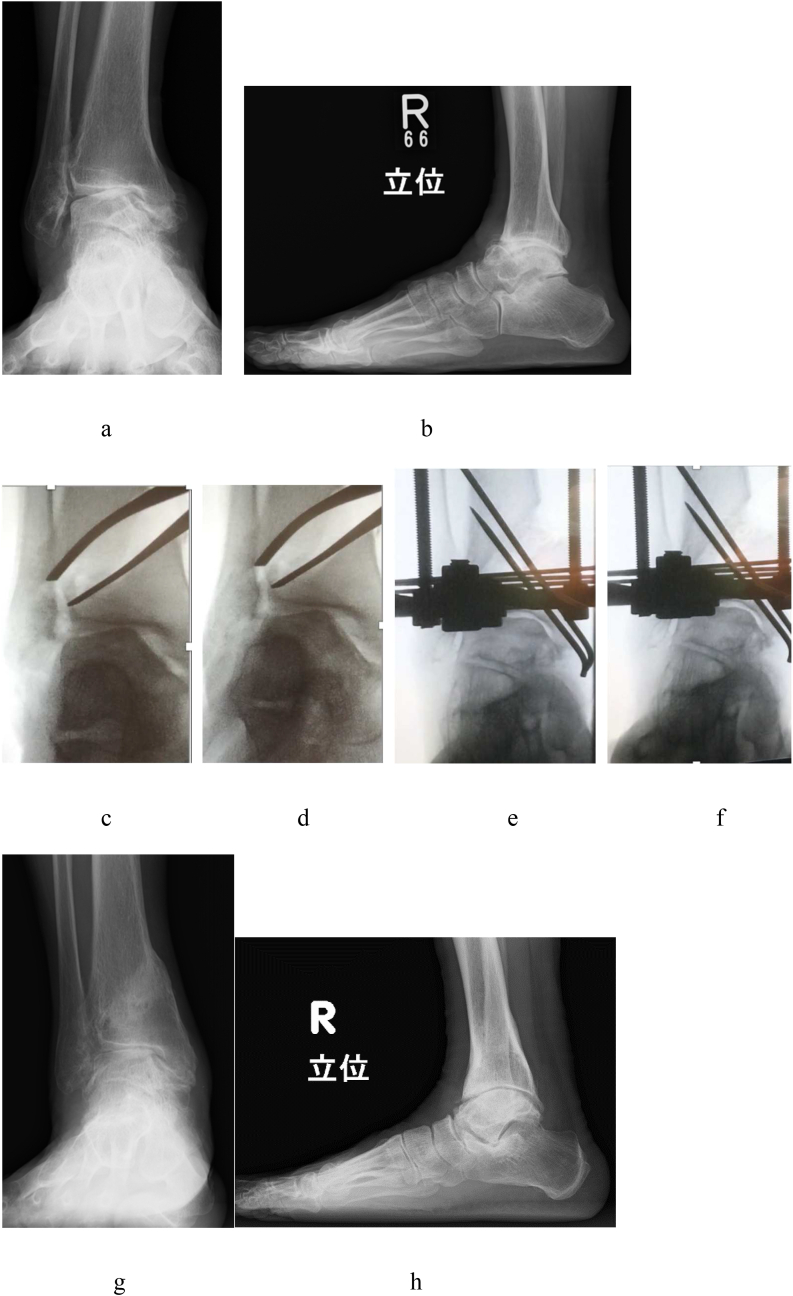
A 69-year-old female. Right ankle osteoarthritis stage 3b (case 2). This is the case of stage 3b without medial talar shift. a. Preoperative weight-bearing ankle radiograph (AP view). The medial plafond is caving in due to the large talar tilt. b. Preoperative weight-bearing ankle radiograph (lateral view). The talocrural joint seems to be unclear due to the anterior subluxation of the talus. c. Intraoperative varus stress fluoroscopy (AP view). d. Intraoperative valgus stress fluoroscopy (AP view). Varus–valgus dynamic instability remains after spreading of the osteotomy gap. e. Postoperative varus stress fluoroscopy (AP view). f. Postoperative valgus stress fluoroscopy (AP view). After lengthening of the Achilles tendon and resection of the bony spurs, dynamic instability seems to disappear. In fact, we change the position of the laminar spreader by trial and error. g. Postoperative weight-bearing ankle radiograph (AP view). The talar tilt is corrected, but the incongruity of the tibial plafond and talar dome seems to remain. h. Postoperative weight-bearing ankle radiograph (lateral view). The anterior subluxation of the talus is corrected, and the congruity of the talocrural joint seems to improve.
